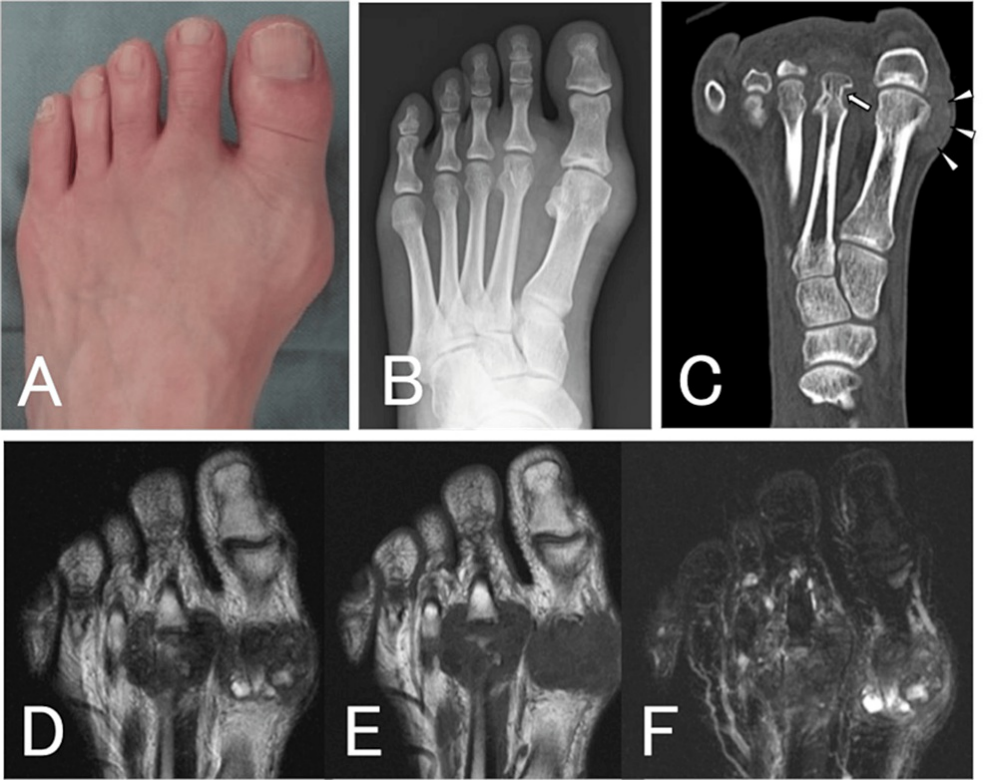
Case two: clinical and radiological findings. A: Photograph taken at the initial examination showing swelling of the metatarsophalangeal joints of the left great and second toes. B: Radiograph showed bony erosions on the heads of the left first and second metatarsals. C: Computed tomography scan showing bony erosion at the neck of the second metatarsal (arrow) and calcification in the soft tissue (arrowheads). D-F: T1-weighted (D) and short tau inversion recovery (F) magnetic resonance images showing a mixed low and high signal, and a T2-weighted image (E) showing a heterogeneous low signal, indicating a mass-like lesion.
